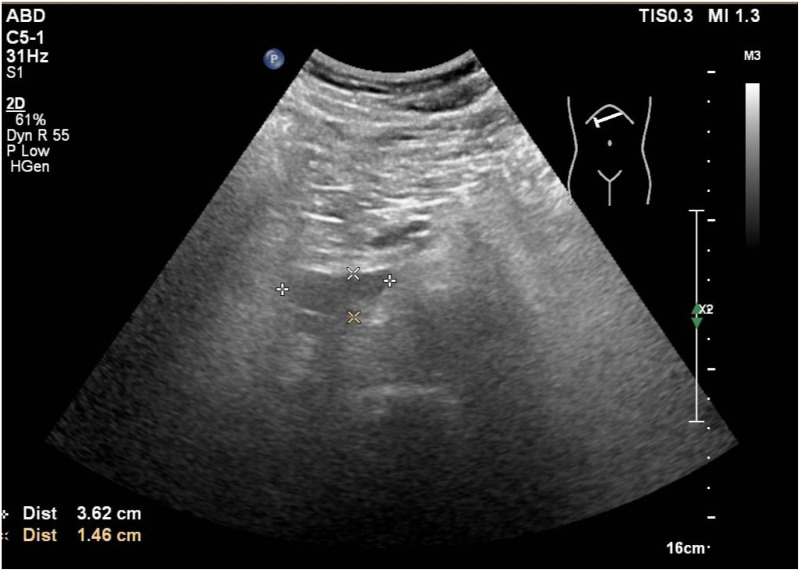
Follow-up ultrasonography of abdominal retroperitoneal lymph nodes after anti-tuberculosis Treatment, and the lymph node mase had a reduction to 3.6×1.5 cm.
